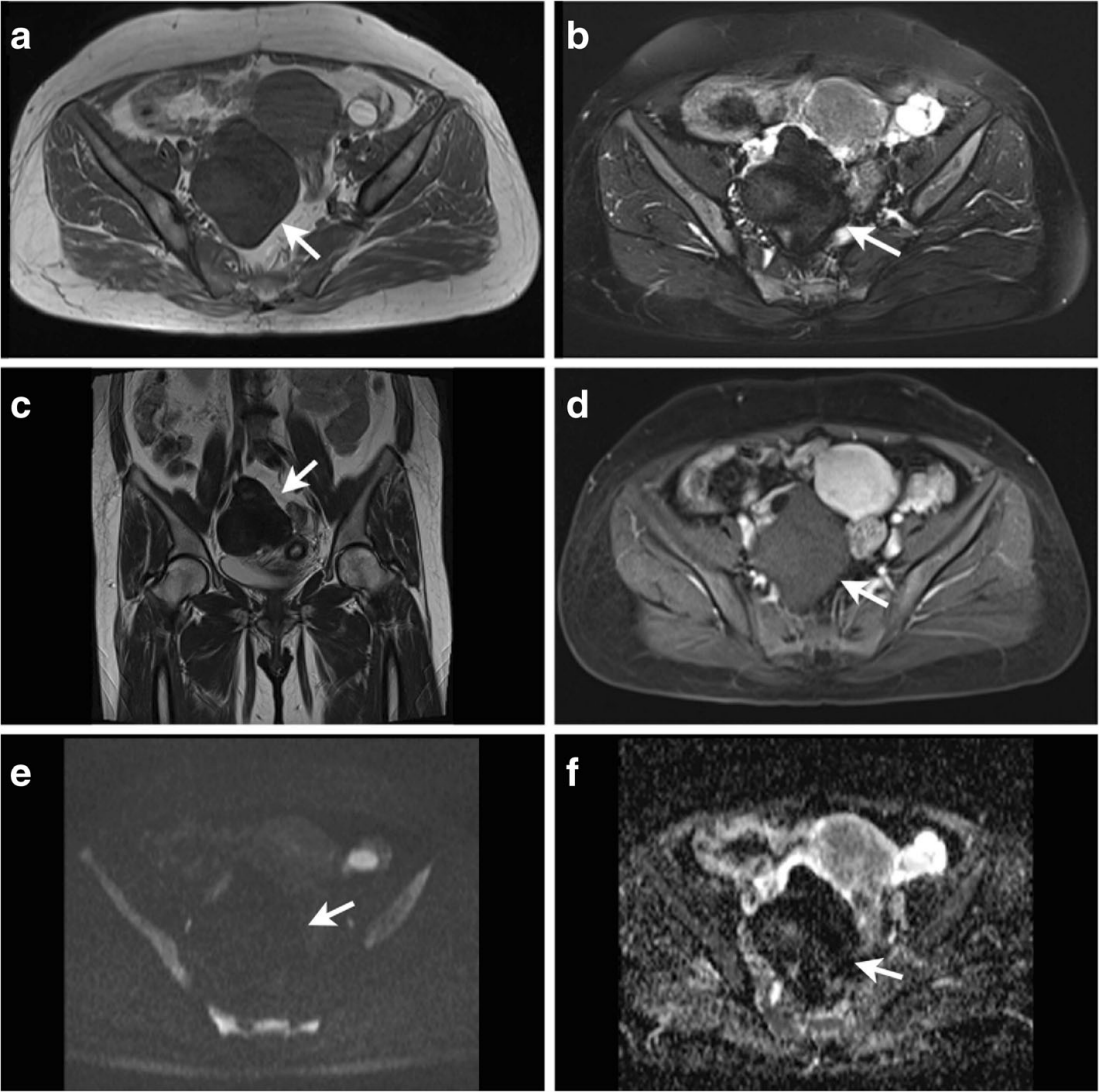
A 47-year-old women with a fibroma on the right ovary(arrows). Axial T1-weighted image (a) shows an oval mass of low signal intensity. Axial T2-weighted with fat suppression image (b) and coronal T2-weighted image (c) shows the mass was homogenous low signal intensity. T1-weighted contrast enhanced images (d) shows the mass was slightly enhanced. The mass shows low signal intensity on DW imaging (e) and has a significant low ADC value of 0.132 × 10^− 3^ mm^2^/s (f)
